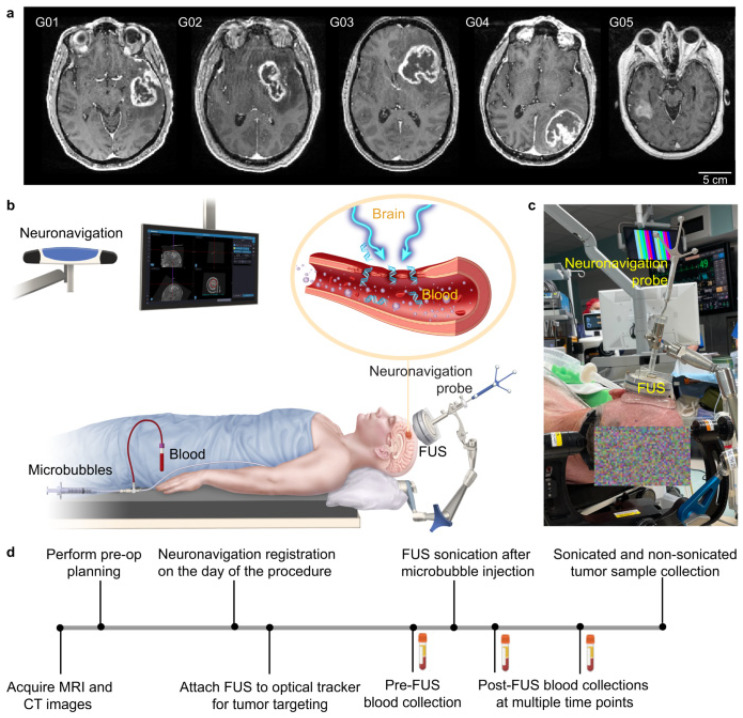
(a) T1-weighted MRI images of the five patients enrolled in the study before sonobiopsy. (b) Picture of the sonobiopsy and neuronavigation system. (c) Image of the FUS transducer paired with a neuronavigation probe used during sonobiopsy. (d) Sonobiopsy procedural steps. Reprinted with permission from Yuan et al. [92].
